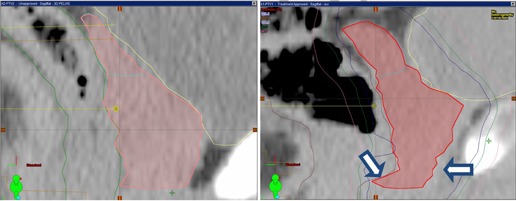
Sample matching result: the new patient CT dataset and prostate segmentation (left panel); the same display for the database case found to be the most similar (right panel). The general prostate shape is similar, with a few discordances visible at arrows. Target inhomogeneity caused by these discordances is solved by running the optimization procedure for a few iterations (shown in Fig. 7).
